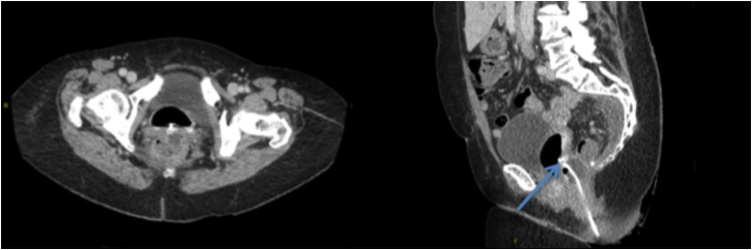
CT scan of the abdomen with rectal contrast shows a rectovaginal fistula. The arrow points to the rectovaginal fistula.
